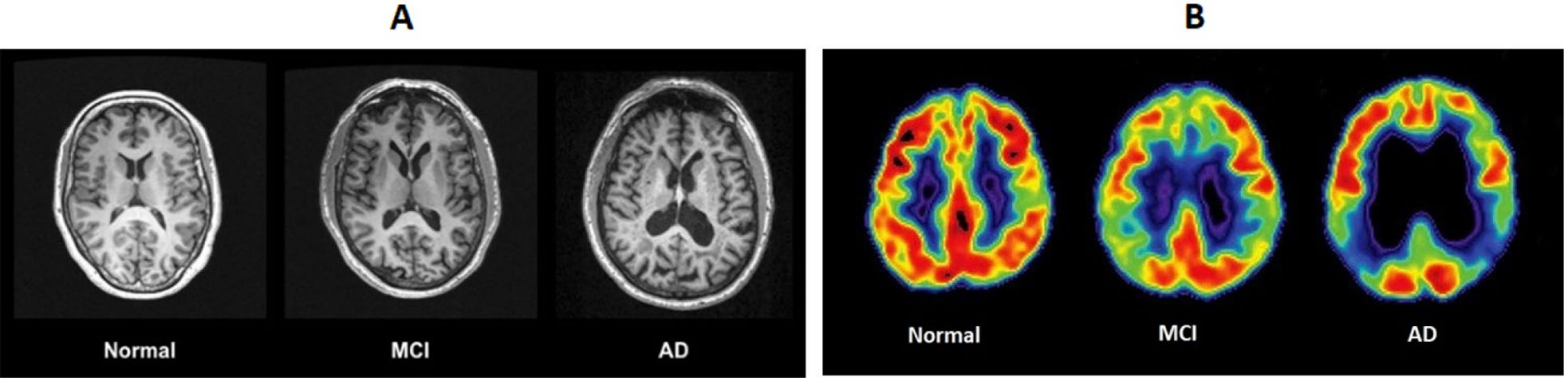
A MRI scans images of different stages of AD. B PET scan images of different stages of AD
